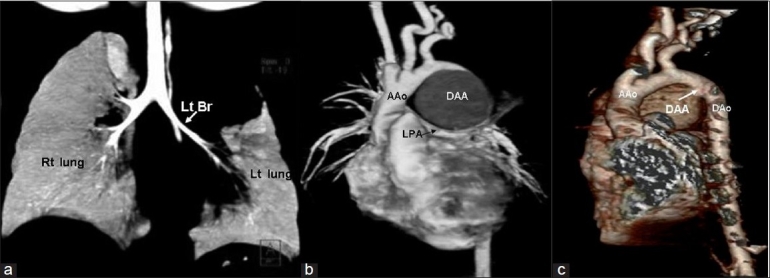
High resolution computed tomography (HRCT) angiography of the chest (a) Compression of left upper lobe bronchus and left upper lobe collapse (b) Large ductal aneurysm compressing the left pulmonary artery (c) Three-dimensional reconstruction of HRCT chest from the posterior aspect, showing the large ductal aneurysm originating from the descending aorta just distal to the origin of the left subclavian artery. Lt Br-left bronchus, rt lung-right lung, lt lung-left lung, AAo-ascending aorta, Dao-descending aorta, LPA-left pulmonary artery, DAA-ductus arteriosus aneurysm
